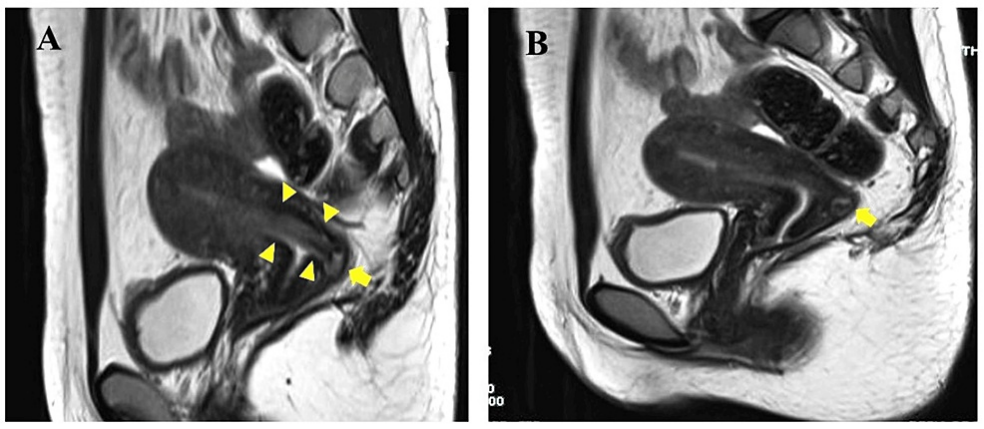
MRI T2-weighted sagittal section of the uterus A: A sticklike image with an iso-intensity in T2-weighted images (arrowheads indicate an artifact in the uterine cavity, arrows indicate a finding suspicious of partial intrusion to the posterior myometrium of the uterus); B: Suspicious finding of partial intrusion to the posterior myometrium (the arrow indicates a finding suspicious of partial intrusion).
